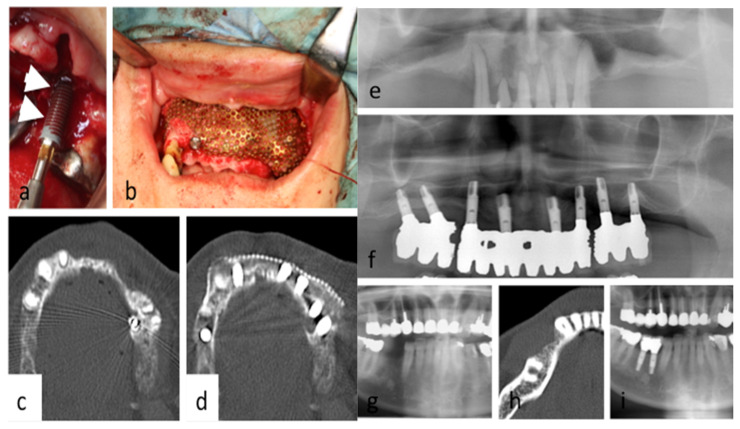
(a) Photofunctionalized implant attracts blood. (b) Simultaneous bone augmentation with PCBM and titanium mesh in atrophied maxilla. Computed tomography before (c) and after (d) placement with simultaneous bone augmentation (a–d; Pt.No.7). Orthopantomography before implantation (e) and after prosthesis (f) (Pt.No.4). Orthopantomography (g) and computed tomography (CT) (h) before implantation. Orthopantomography (i) five years after final prosthesis (g–i; Pt.No.2).
